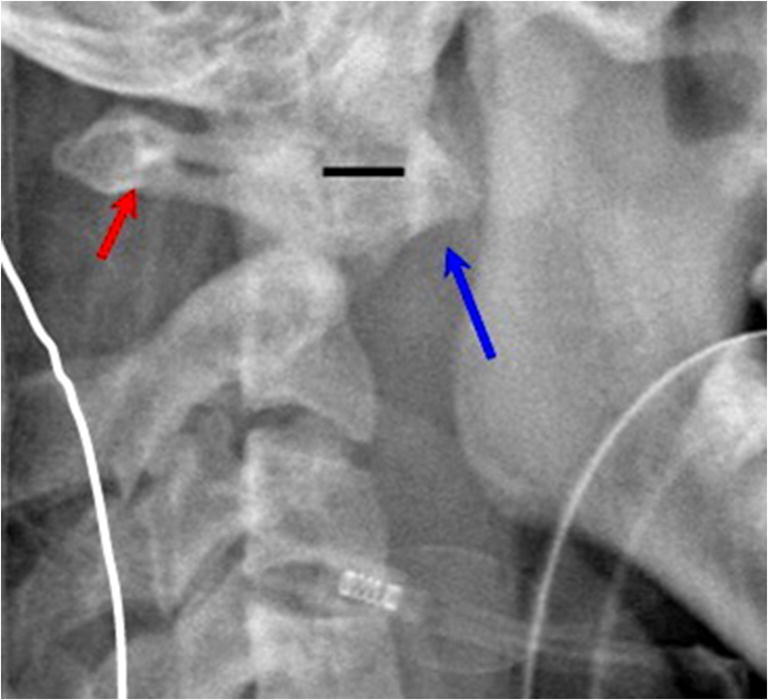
Lateral cervical spine x-ray demonstrates atlantoaxial instability (AAI) in Down syndrome. In this case, mild flexion of the neck demonstrates a widened atlanto-dental interval (solid bar) between the anterior tubercle of C1 (long arrow) and the odontoid process that exceeds the pathological threshold in the adult of 3 mm. The spinal canal (small arrow) is substantially diminished and results in spinal cord compression
